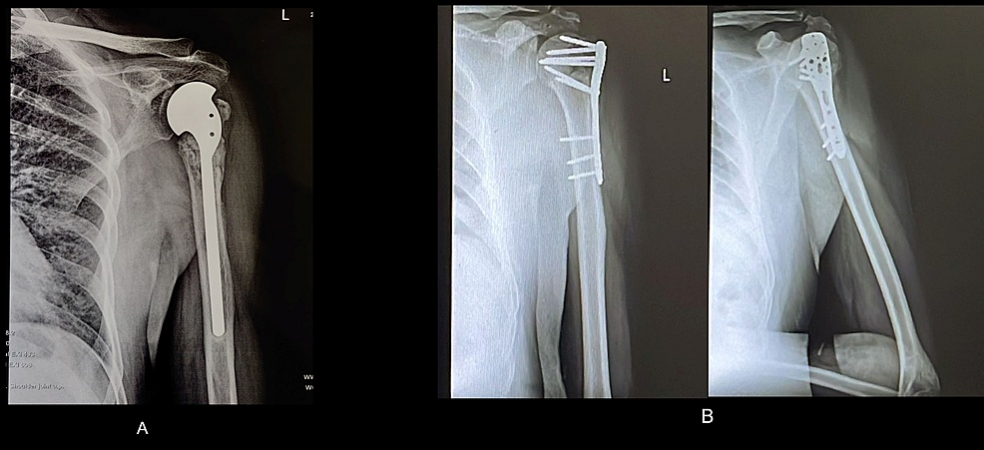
Two years post-operative follow-up X-ray of Neer's hemiarthroplasty and proximal humerus internal locking system (PHILOS) plating
